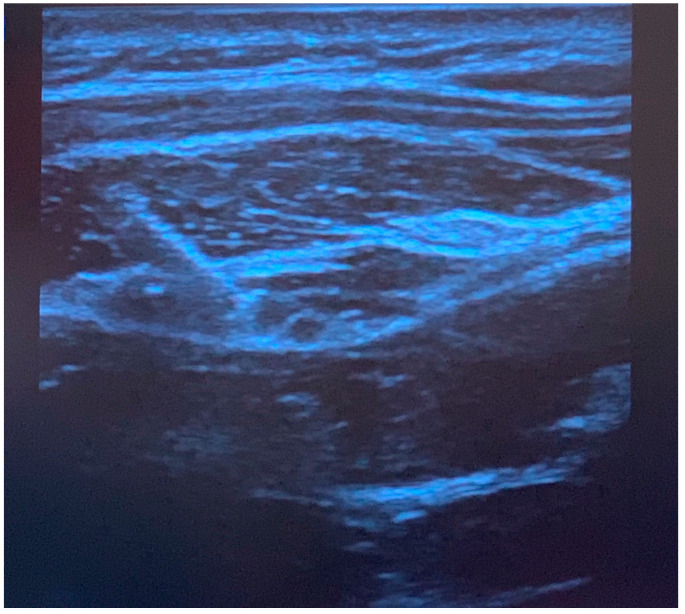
Ultrasound image of greater occipital nerve block injection. The needle is positioned superior to the obliquus capitis inferior muscle, and local anesthetic spread is observed in the fascial plane. The semispinalis muscle is observed above the fascia.
